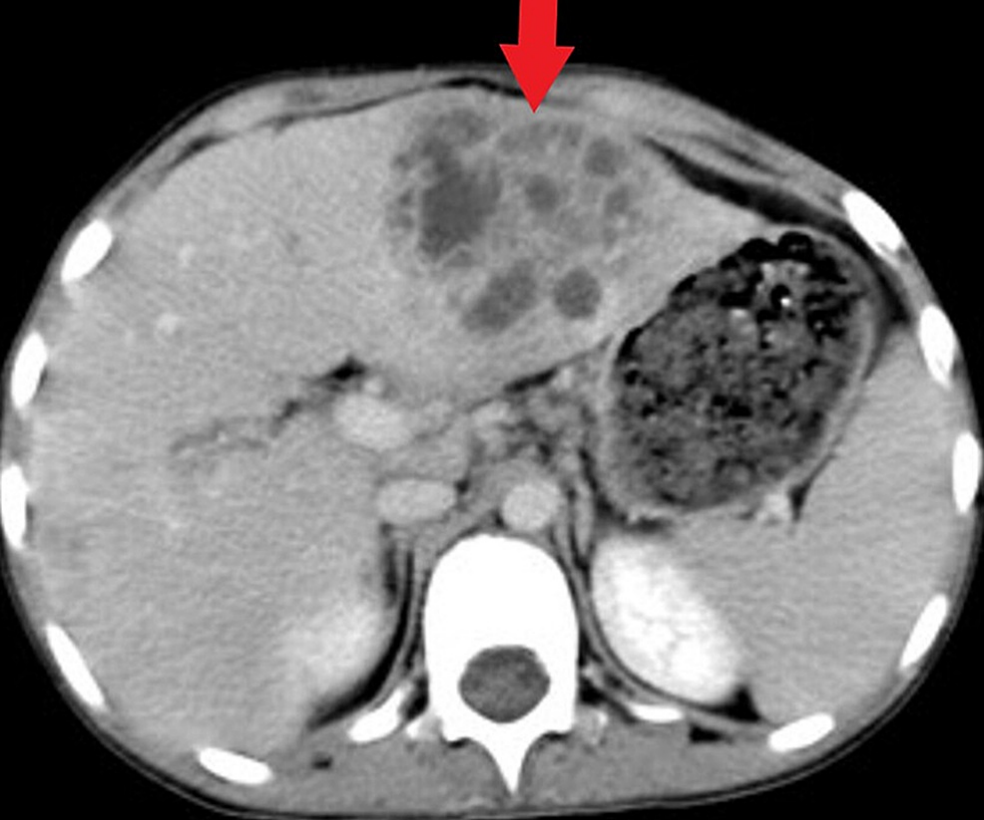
CT scan of the abdomen, axial plane with no contrast showing multiple cystic lesions in the left liver lobe (subcapsular in location) grape-like suggestive of hepatic abscesses.
